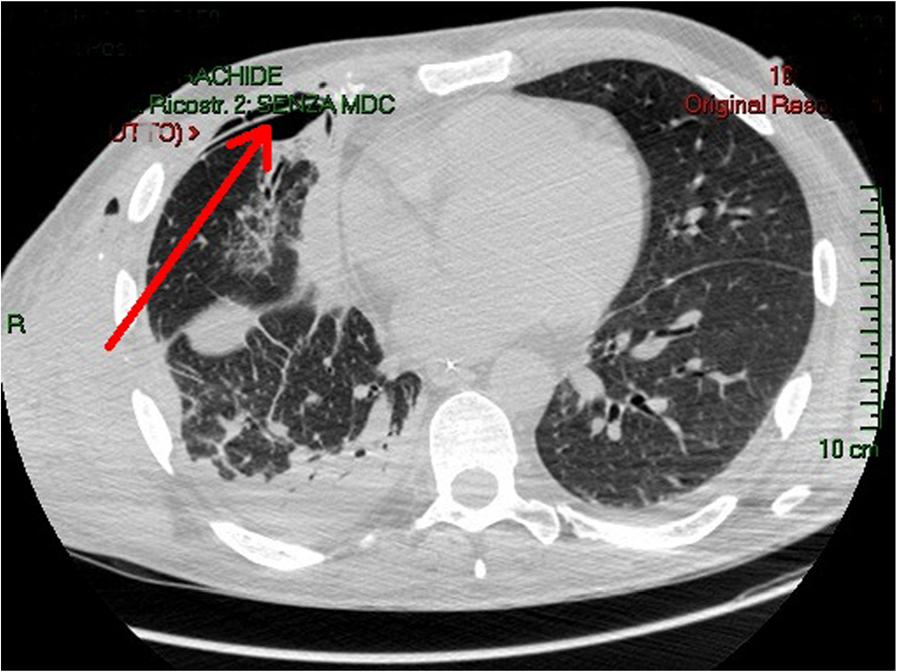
CT-scan 9 days after the reconstruction; the red arrow indicates the prosthesis.
